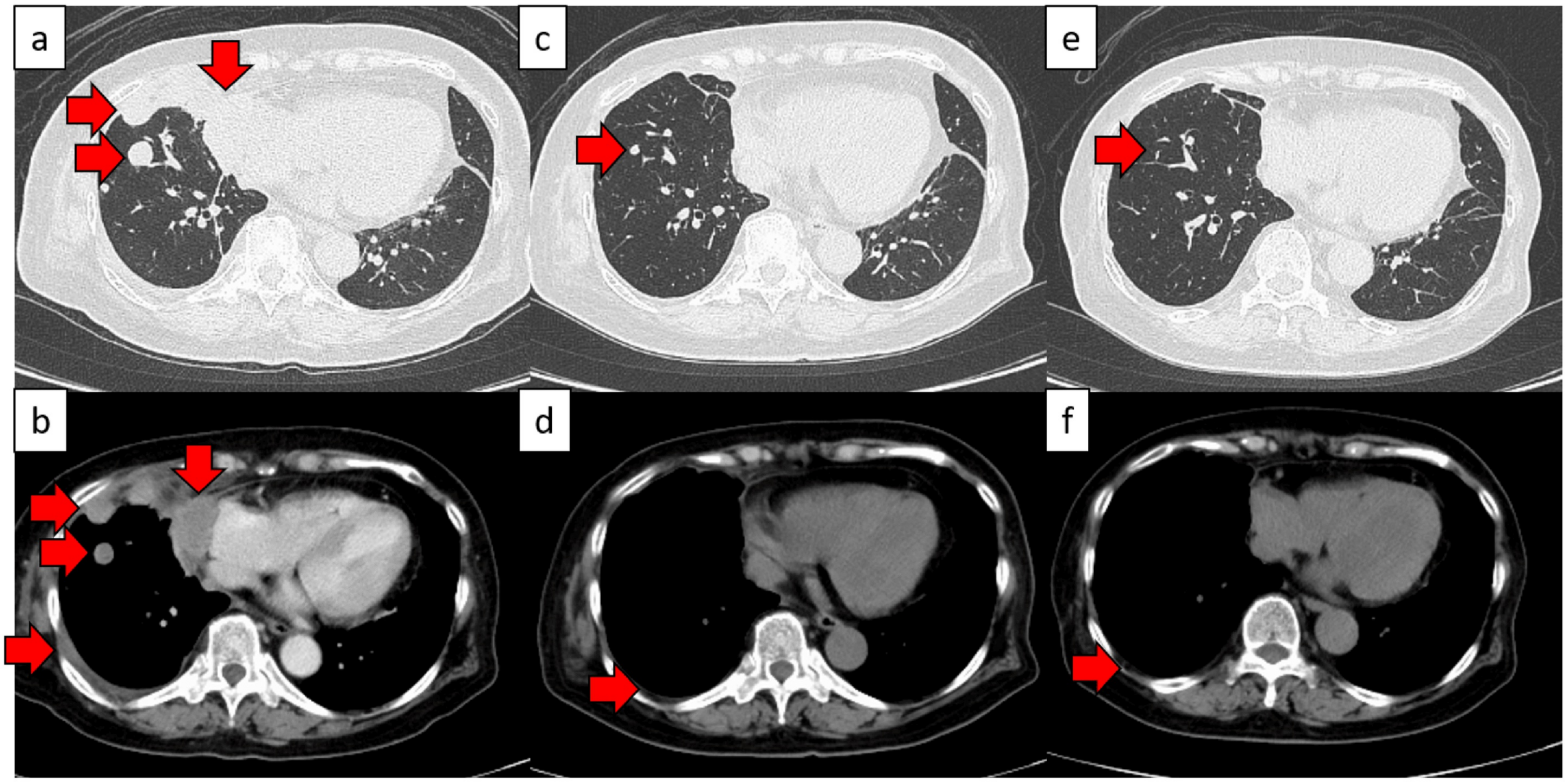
CT results before and after chemotherapeutic treatment (a, b) CT scans before chemotherapy. Lung metastasis and pleural dissemination were observed. ➡ Lung metastasis and pleural dissemination (c, d) CT scans three months after the start of chemotherapy. (c) The metastatic lung tumor and pleural dissemination tended to shrink. ➡ Metastatic lung tumor exhibiting a tendency to shrink. (d) Reduced right pleural effusion was observed. ➡ Reduced right pleural effusion (e, f) CT scans six months after the beginning of chemotherapy. (e) The metastatic lung tumor disappeared, and complete remission was considered to be achieved. ➡ Disappearance of the metastatic lung tumor. (f) The right pleural effusion disappeared. ➡ Disappearance of the right pleural effusion
